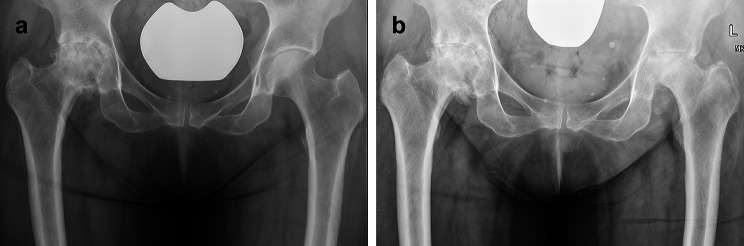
Radiographs of the pelvis of a 60-year-old female in antero-posterior view. (a) Radiograph prior to ICSI to the left hip: The left hip shows joint space narrowing and subchondral sclerosis. The right hip shows severe OA with multiple osteophytes, subchondral cysts, and joint space narrowing. Despite the radiographic findings, the right hip was clinically less symptomatic than the left hip. (b) Radiograph 4 months after ICSI to the left hip. There are no radiographic changes in the right hip. The left hip shows RPIO with bone-to-bone OA and severe bone loss of the femoral head
